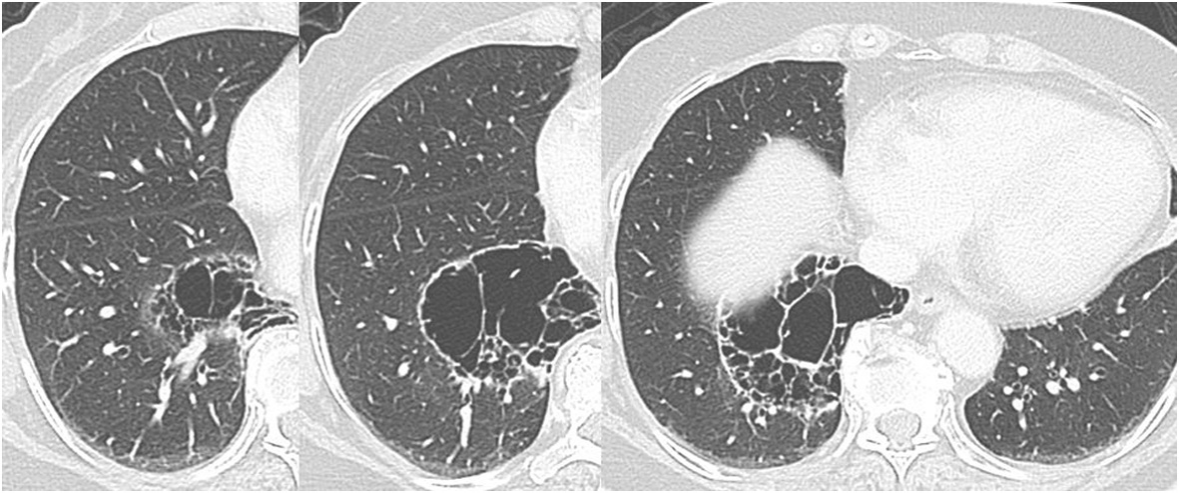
Chest computed tomographic images show bullous emphysema in the right lower lobe. There is no aberrant artery and no communication with the bronchus.
